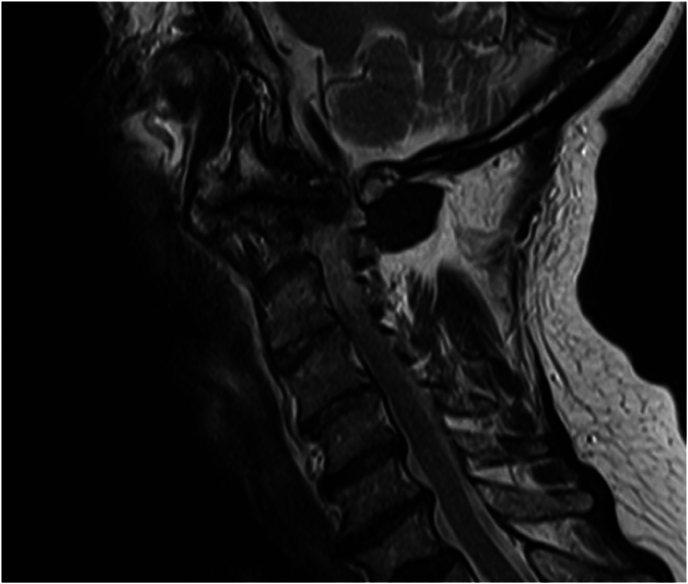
T2 MRI sagittal cervical spine where blossoming of the facet joint can be observed. T2 MRI sagittal cervical spine where blossoming of the facet joint can be observed.
